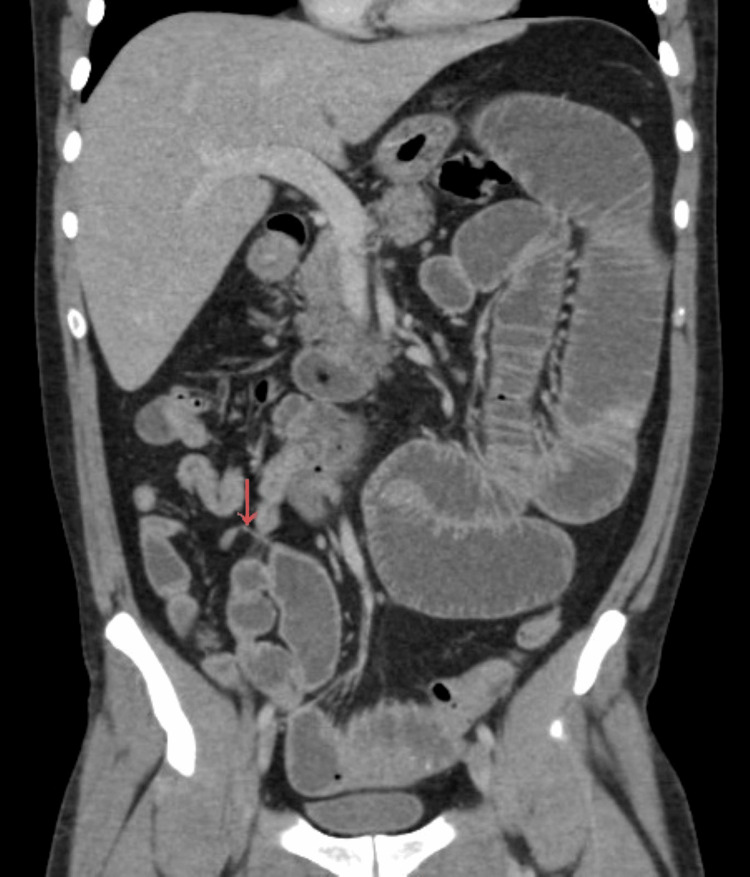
CT identification of a feeding vessel associated with Meckel’s diverticulum. Contrast-enhanced CT image showing a prominent vessel supplying the diverticulum (arrow), a radiologic feature that supports the diagnosis of Meckel’s diverticulum.
